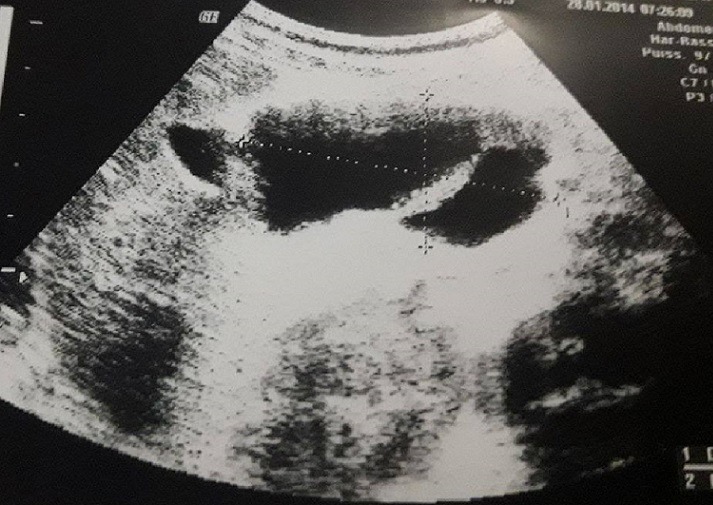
Ultrasound image showing a hepatic hilar multilocular cystic mass in contact with the anterior surface of the head of the pancreas
